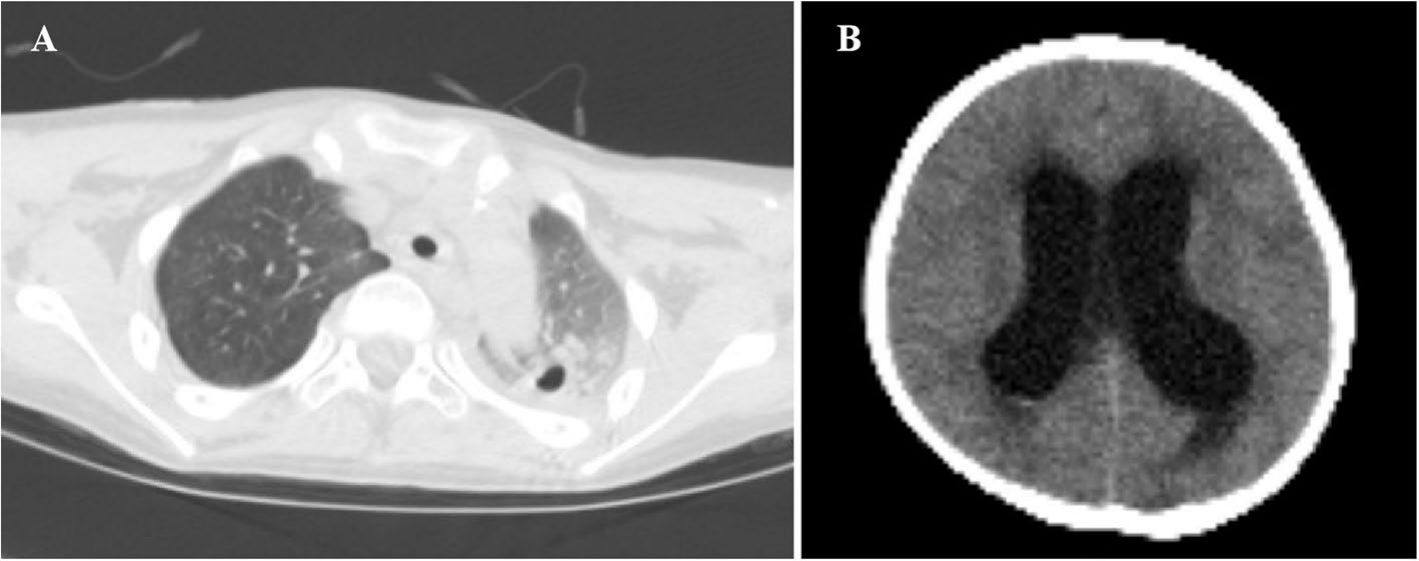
CT scans of Patient 1 with p67phox-deficient CGD, demonstrating cavitary pneumonia (Panel A) and communicating hydrocephalus (Panel B)
